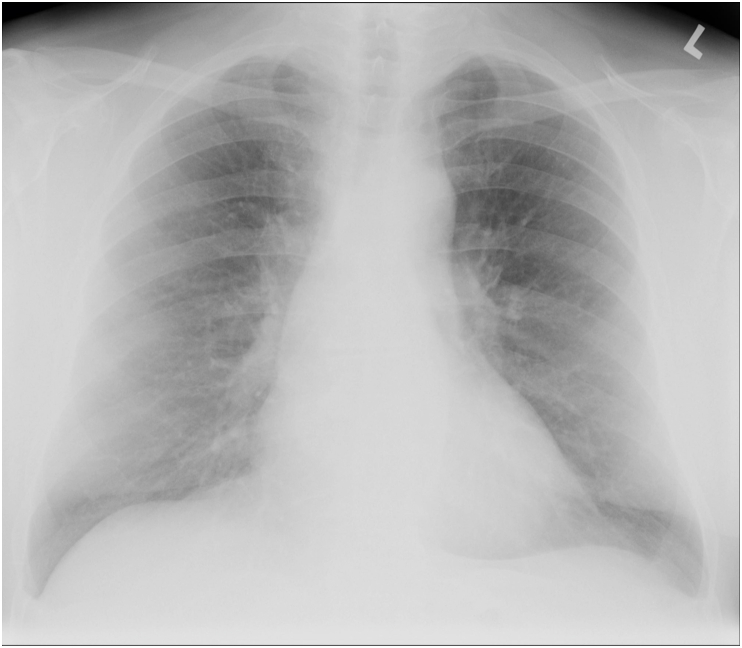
Preoperative chest x-ray – no visible metastasis.
